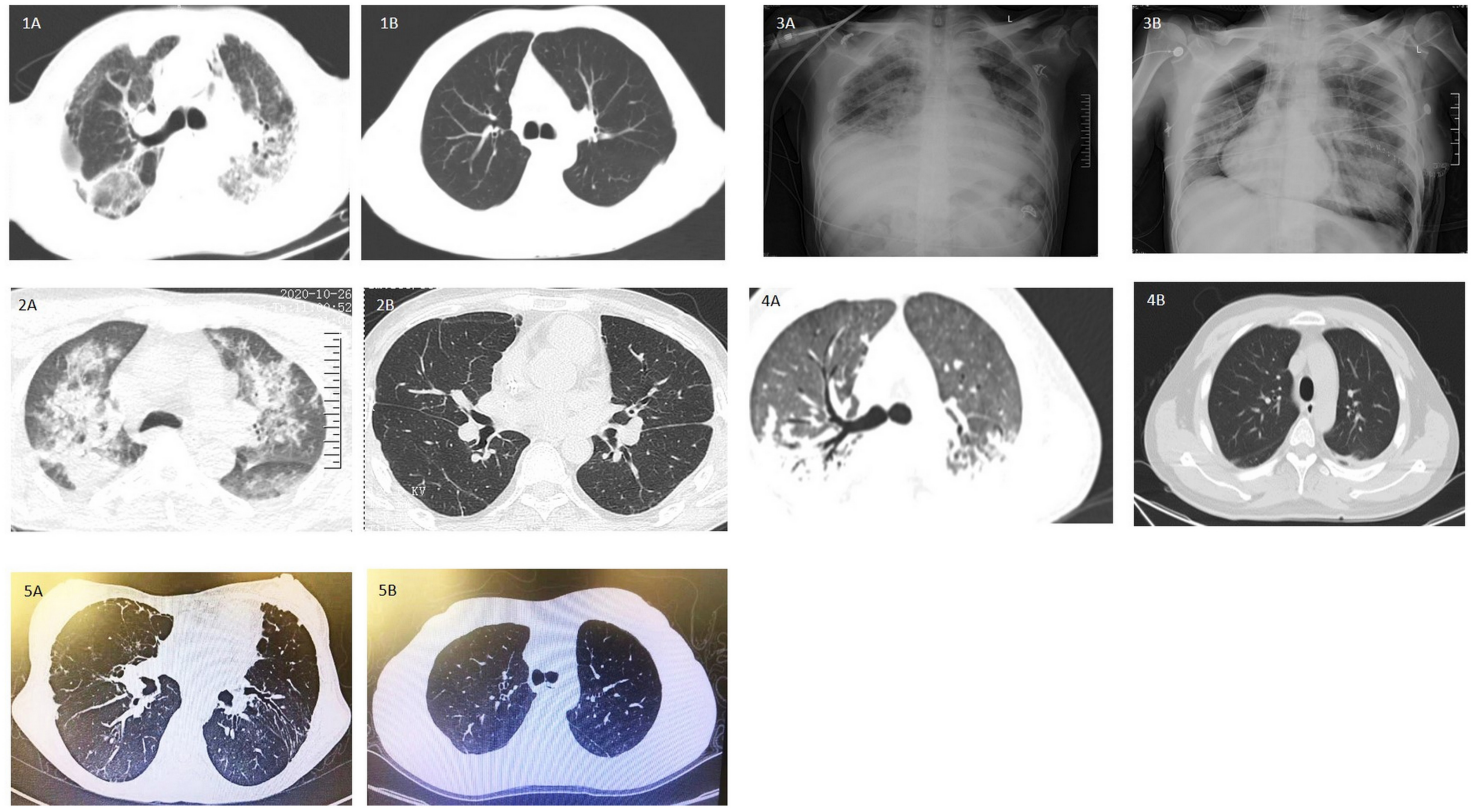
CT images of five patients pre-lung transplantation and post-lung transplantation. (1A−4A) Progression of bilateral lung fibrosis with consolidation were shown in case 1–4. (1B, 2B, 4B, 5B) Post-lung transplantation CT manifestation without significant abnormality. (3B) Chest imaging showed significant lung effusion and edema in Case 3. (5A) Case 5 suffered deteriorated graft function after 5 years of transplantation and chronic lung allograft dysfunction was suspected.
